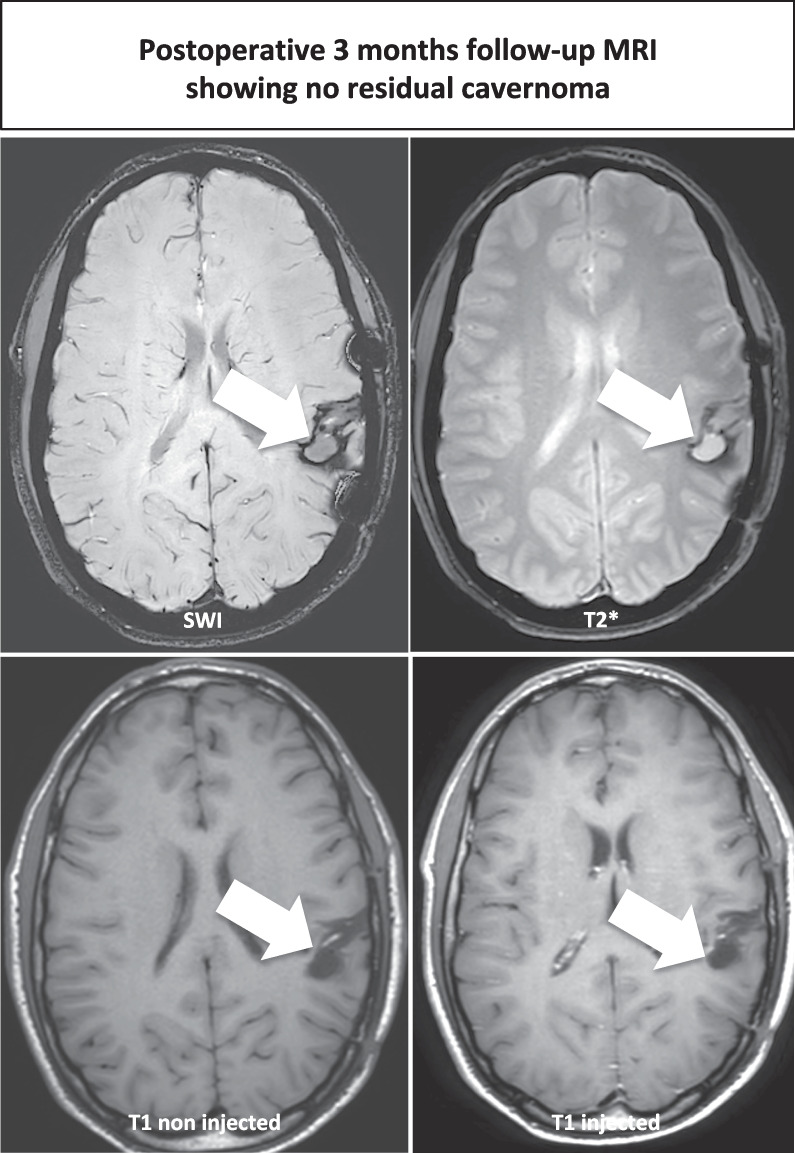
Postoperative MRI assessment. The arrows point out the cavernoma
